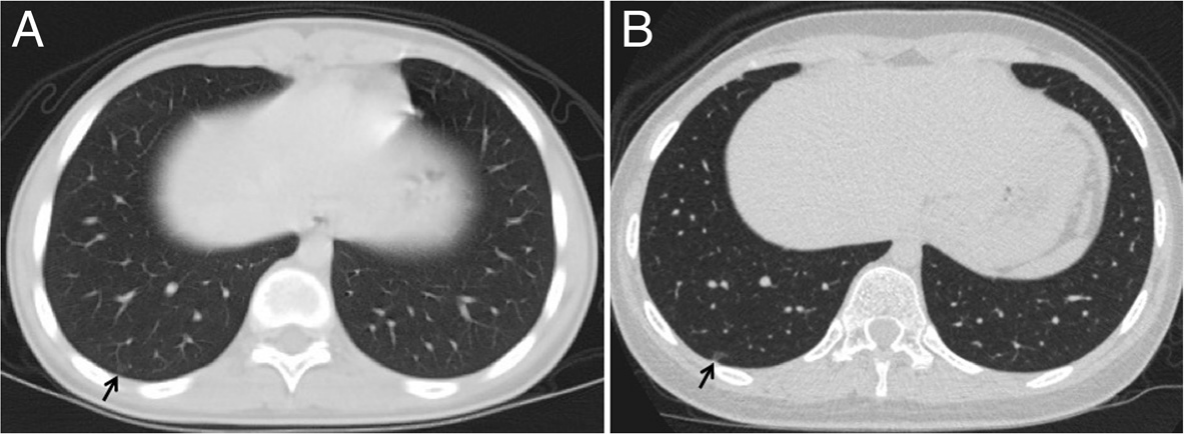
Radiological findings of bronchioloalveolar carcinoma. (A) Chest CT just before the left tibia surgery showed an extremely faint, small shadow in right S9. (B) When the patient was referred to our department 1 year later, chest CT revealed a 3-mm GGO at the same site.
